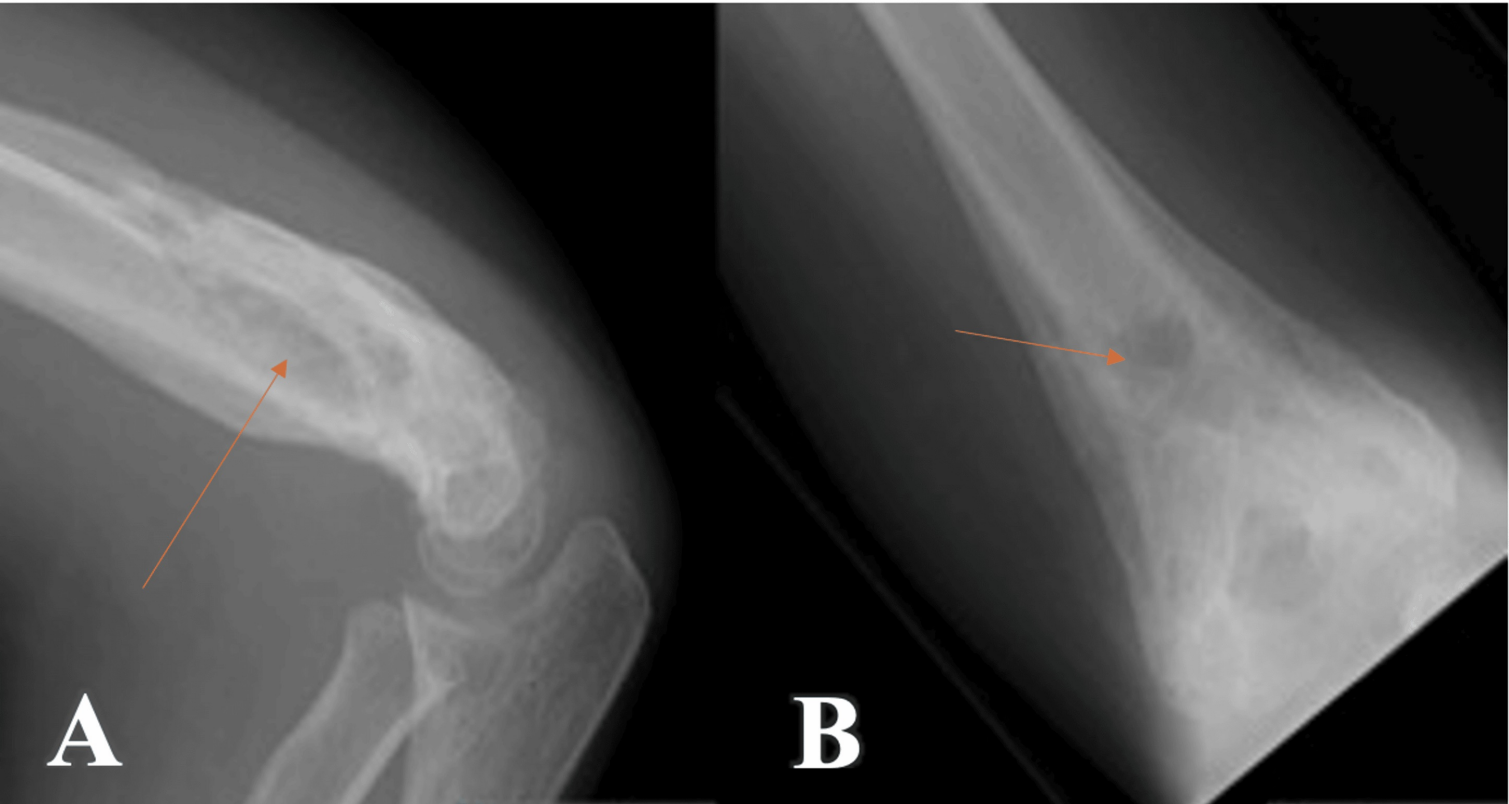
10 weeks postoperative radiograph, with radiological signs of deep infection (excessive periosteal reaction, with central bone cyst) A - Lateral view of the elbow; B - Anteroposterior view of the elbow
